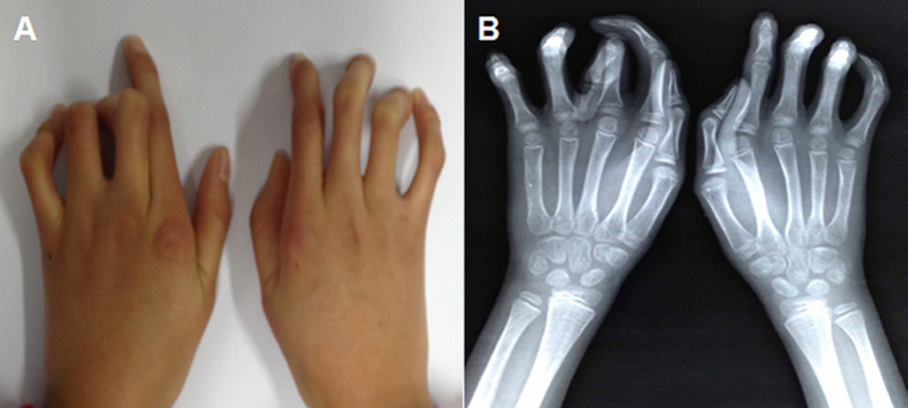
(A) The phenotype and (B) X-ray images of hands from an affected member (IV:2) of the family.
